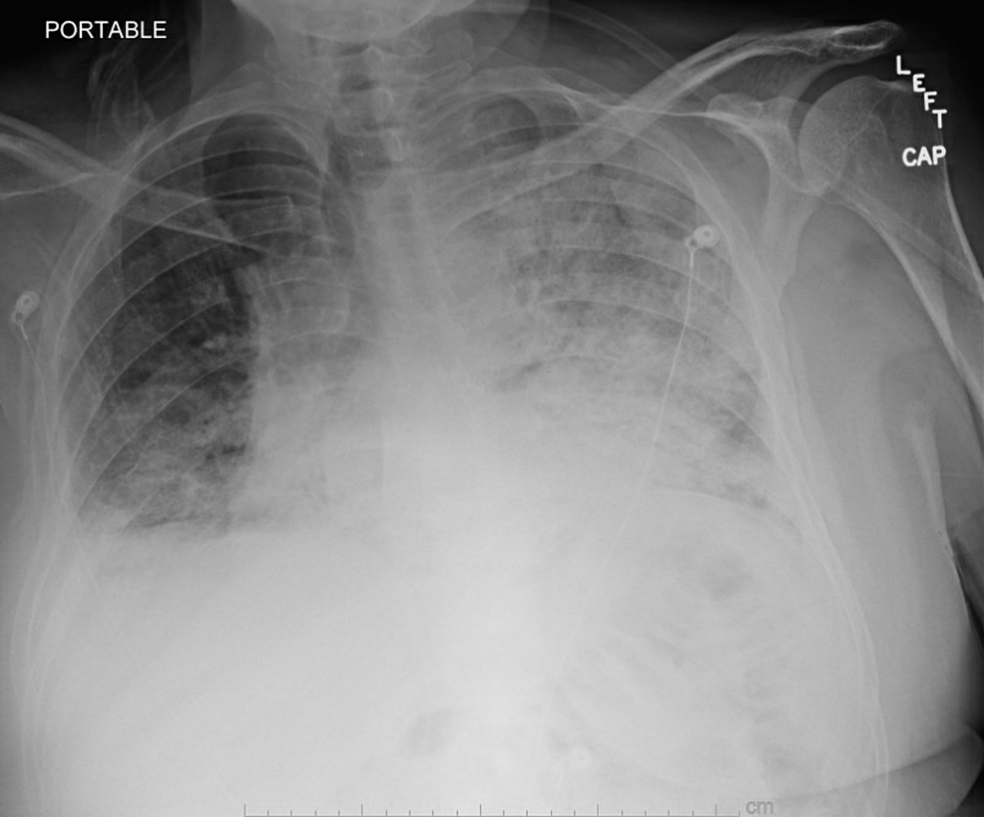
Chest X-ray performed four days after admission This was performed due to the patient's increased oxygen requirements. There is a significant worsening of the bilateral infiltrates, with more on the left compared to the right.
